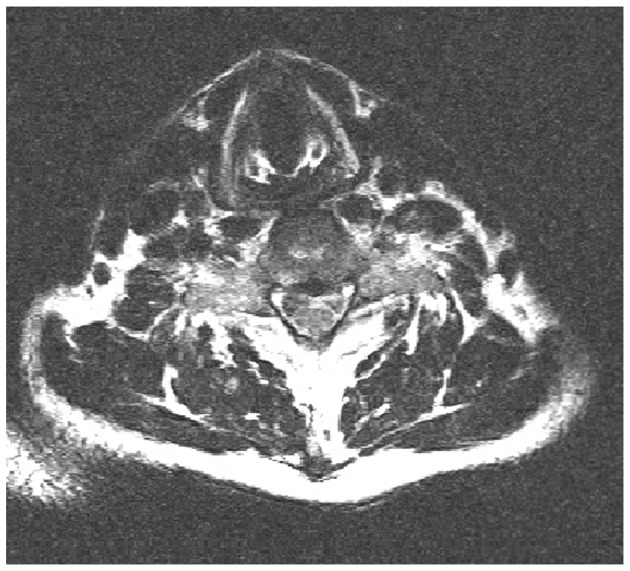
Magnetic resonance imaging demonstrating compression of the C6 vertebral body of the spinal cord.
